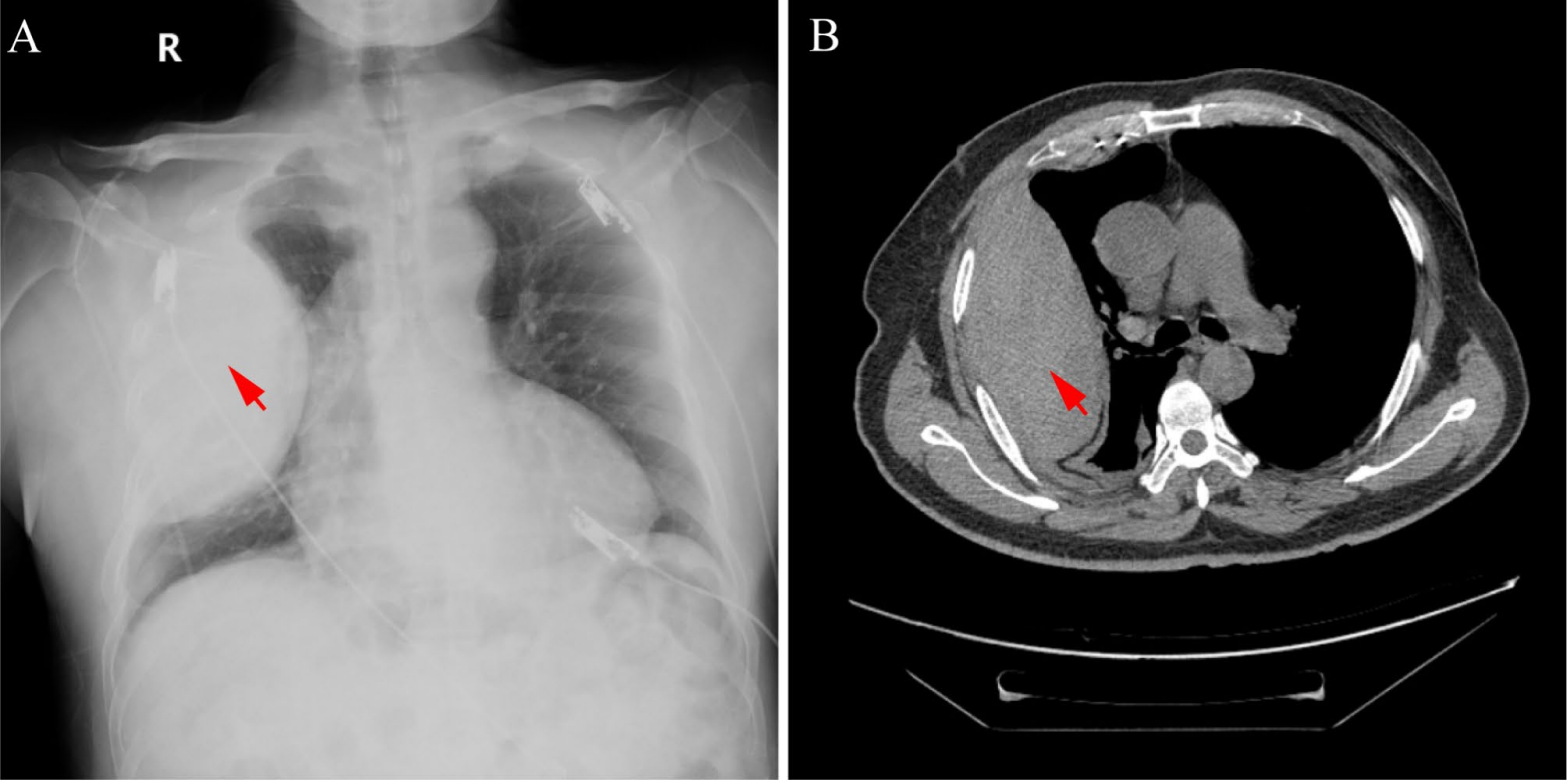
Radiographic images of encapsulated pleural effusion (red arrow)
